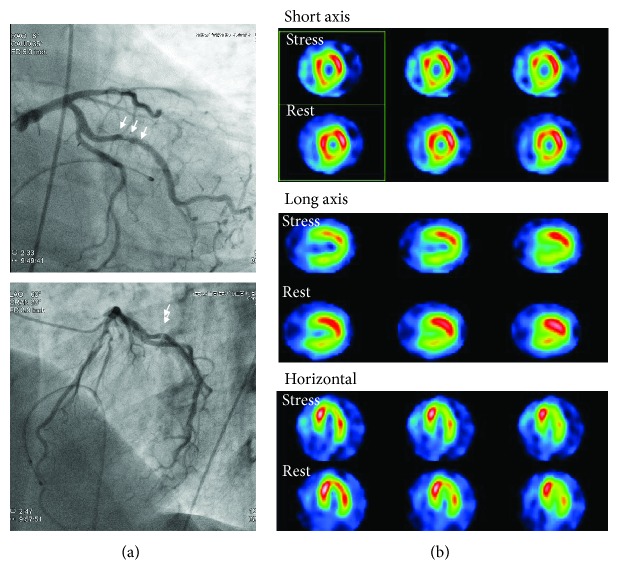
Second CAG and adenosine stress myocardial perfusion scintigraphy. (a) The second CAG was performed to investigate the acetylcholine-induced vasospasm. Although the coronary arteries did not exhibit vasospasm induced by acetylcholine, CAG revealed de novo stenosis in #12. (b) The adenosine stress myocardial perfusion scintigraphy (Tc-99m tetrofosmin) showed the myocardial ischemia in the posterior wall area, suggesting myocardial ischemia in #12.
